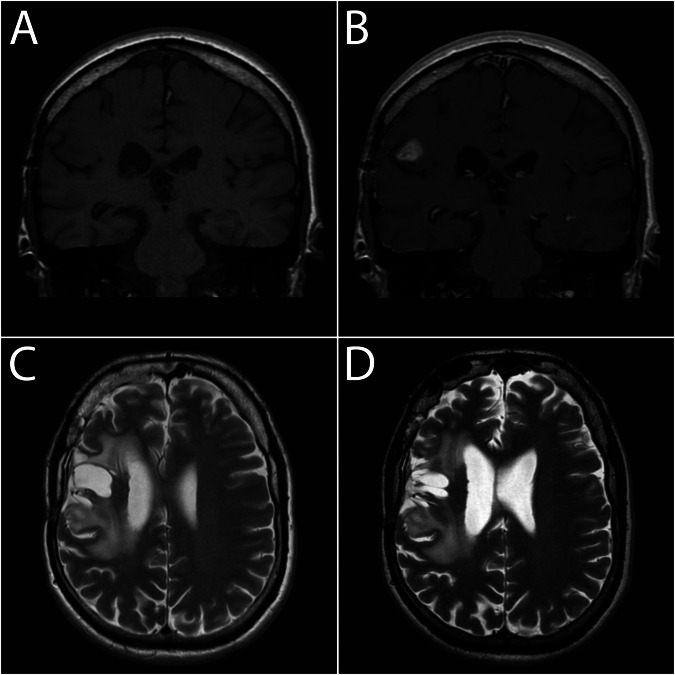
Effect of GBCA on T1- and T2-weighted images: A precontrast T1-weighted image, (B) postcontrast T1-weighted image with postcontrast enhancement, (C) precontrast T2-weighted image, and (D) postcontrast T2-weighted images. No signs of T2 postcontrast enhancement are present, although as seen in (B) the blood-brain barrier is impaired
